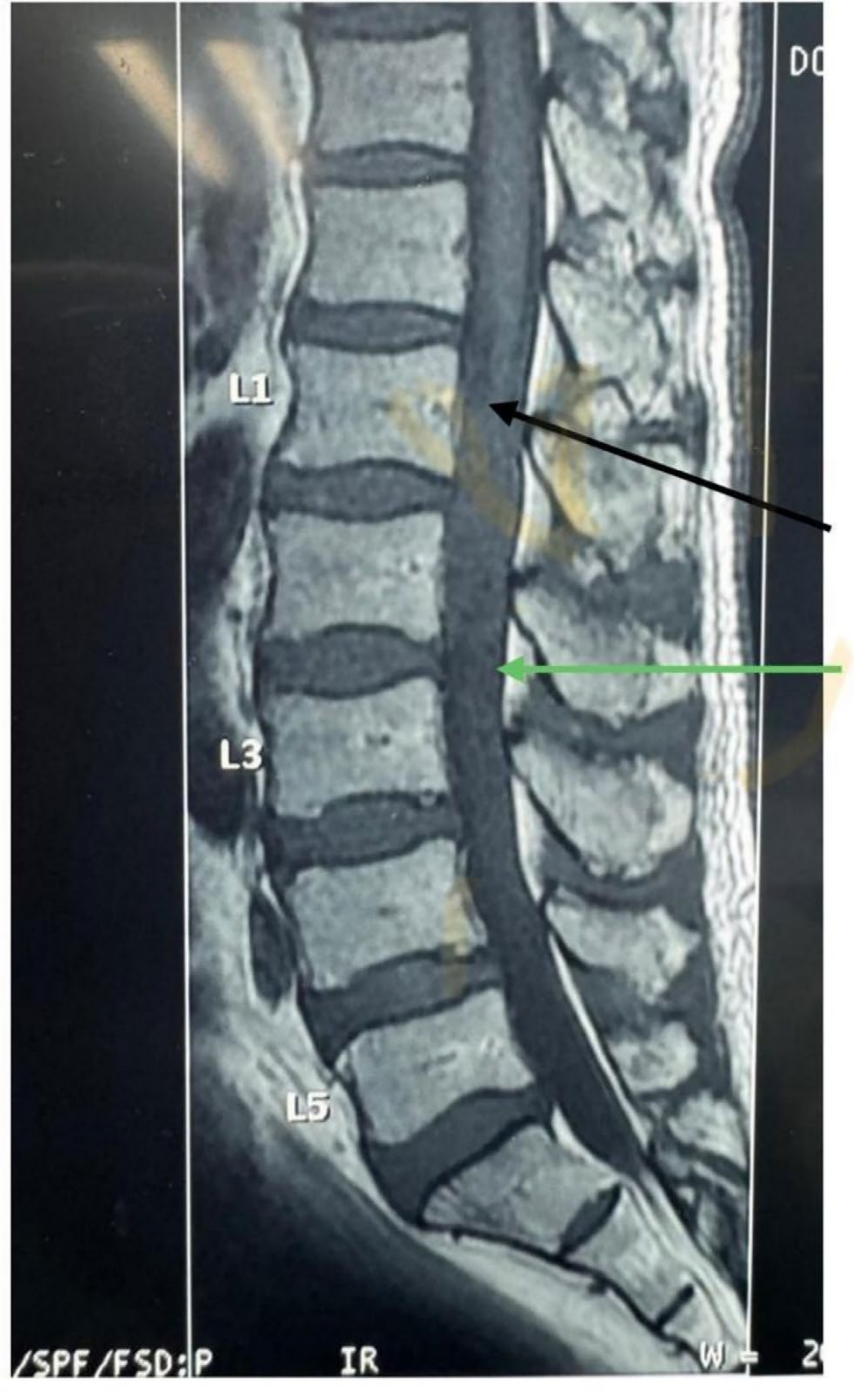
Pre‐operative magnetic resonance imaging T1 weighted image shows a 4.33 cm craniocaudal, 1.60 cm transverse, and 1.0 cm AP diameter, well‐defined, sharply marginated oval shape, isointense intradural extramedullary mass extending from L1 to L2. It fills the spinal canal. The arrows show an isointense intramedullary spinal cord tumour. The black arrow shows the proximal extent/border while the green arrow shows the caudal extent.
